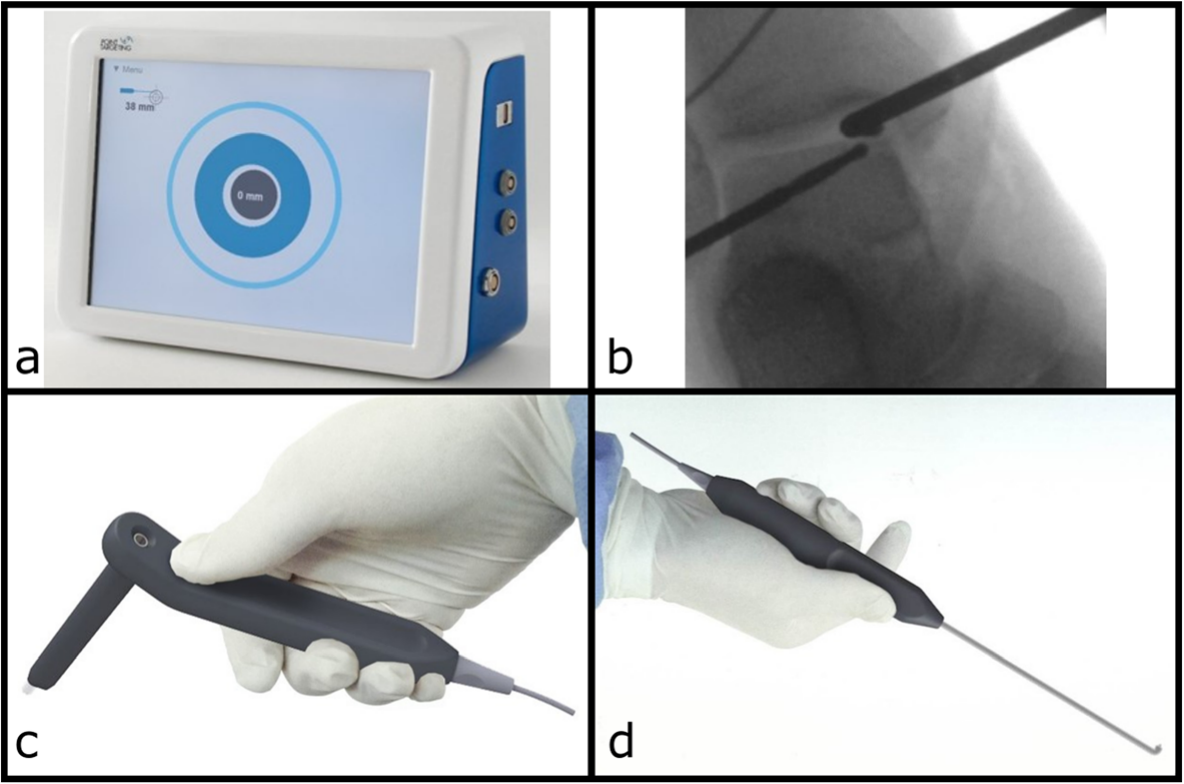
a/b/c/d Intraoperative equipment: aMonitor. b Intraoperative x-ray. c Drill sleeve. d Probe hook
